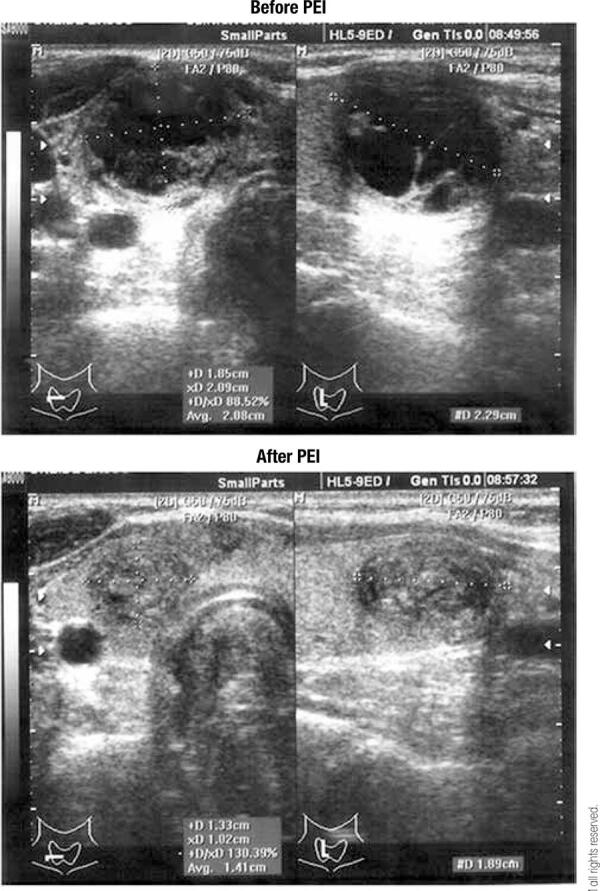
Sequence of ultrasonographic images before and after percutaneous ethanol injection (PEI, above and below images, respectively). The volume of the nodule before treatment was 4.5 cm3 and after treatment was 1.3 cm3.
